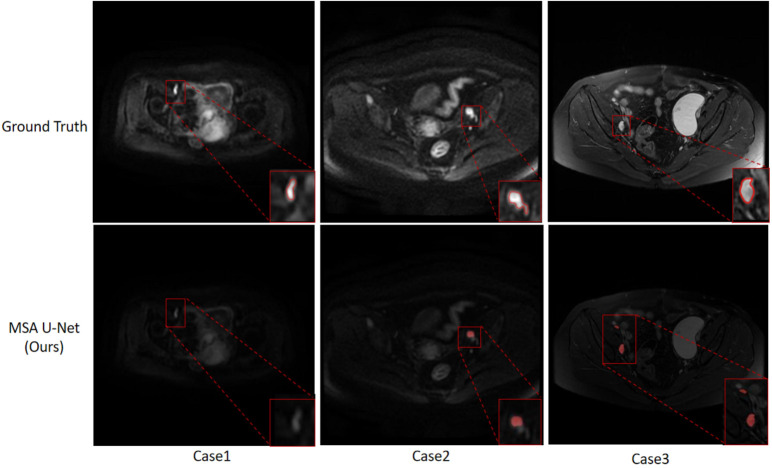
Representative failure cases of MSA U-Net. Case 1: missed segmentation of a LN. Case 2: under-segmentation caused by indistinct lesion boundaries. Case 3: over-segmentation due to confusion with surrounding tissues exhibiting similar MRI appearances
